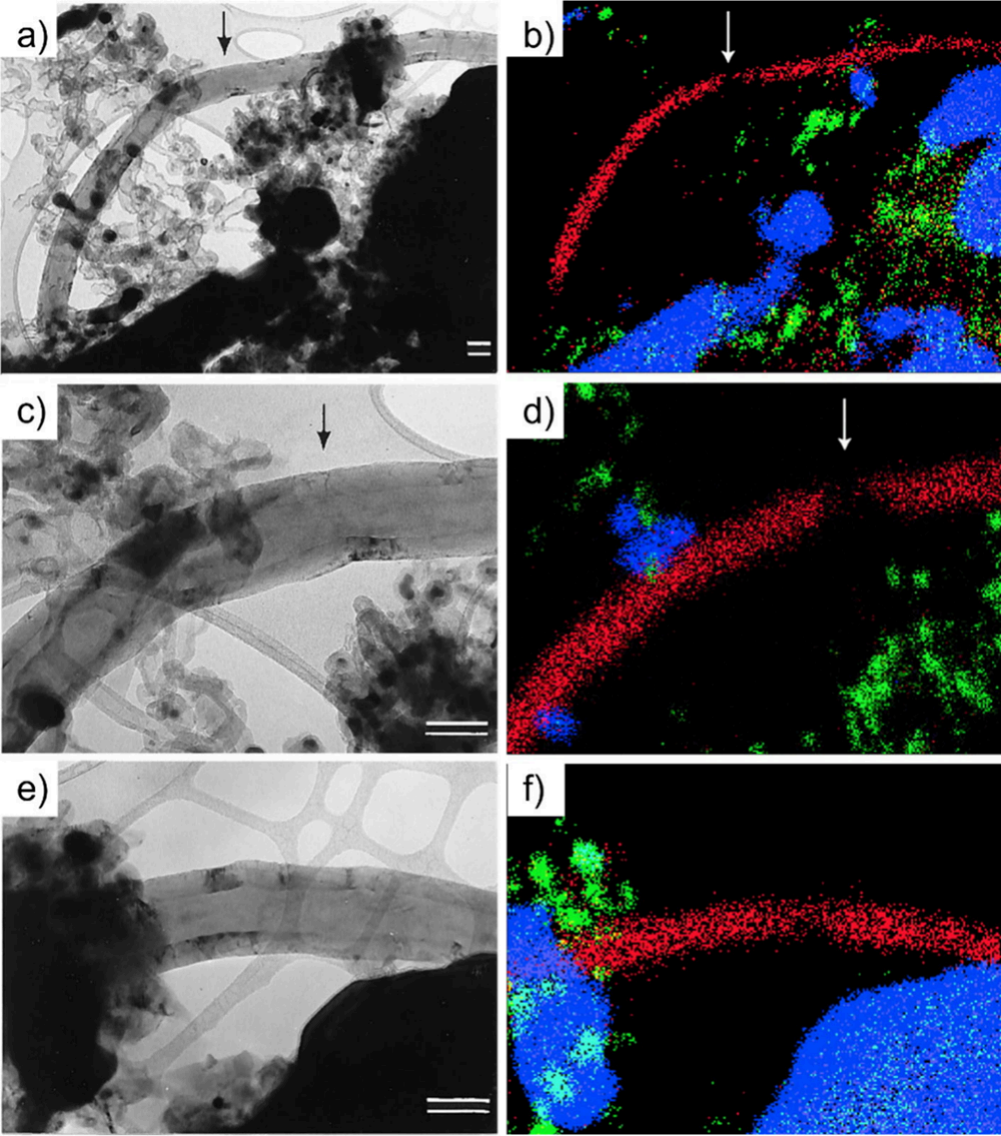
TEM images and X-ray maps of a typical Ar-filled CNT that winds
